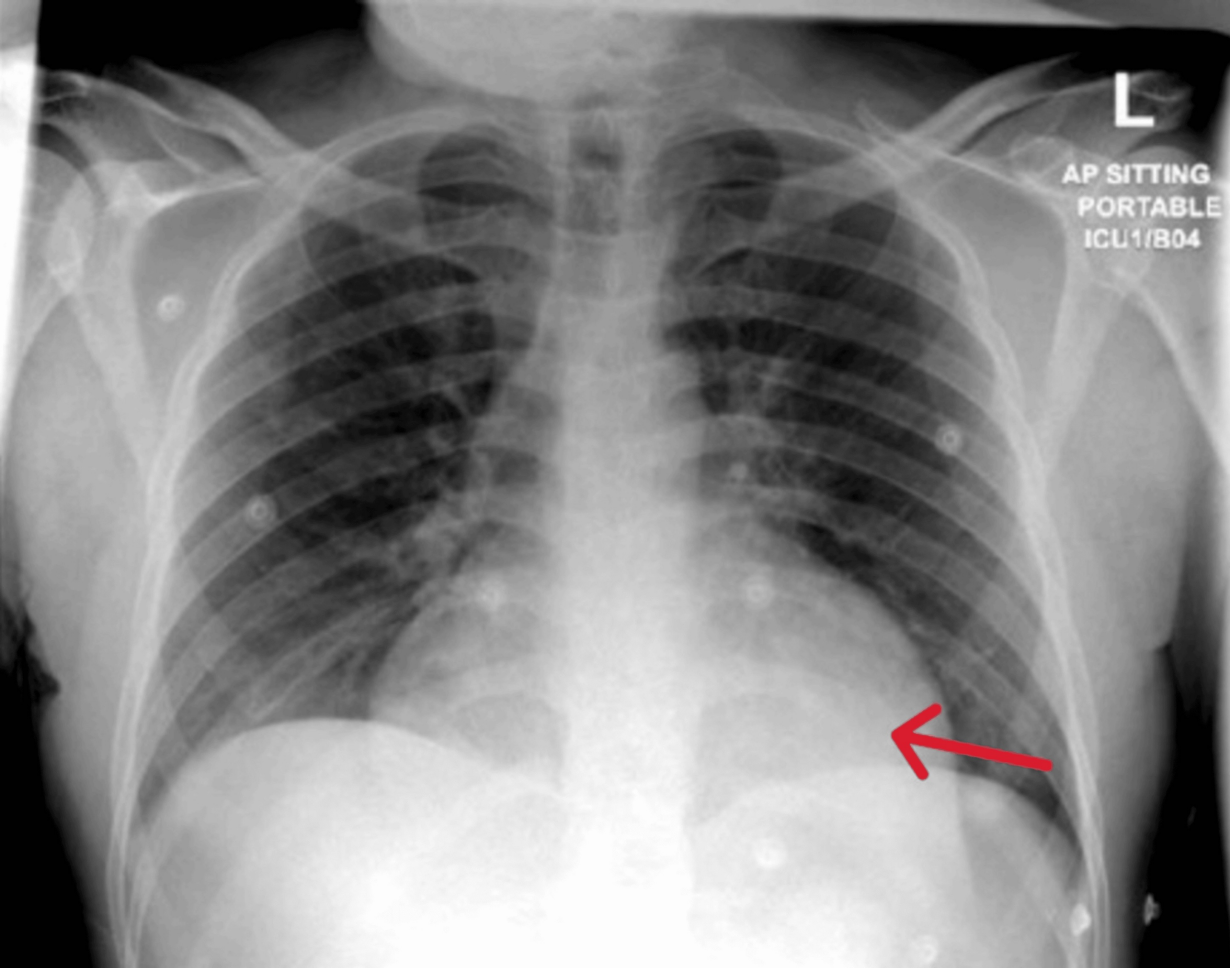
Chest X-ray, posteroanterior view, showing marked enlargement of the cardiac silhouette consistent with cardiomegaly (red arrow)
